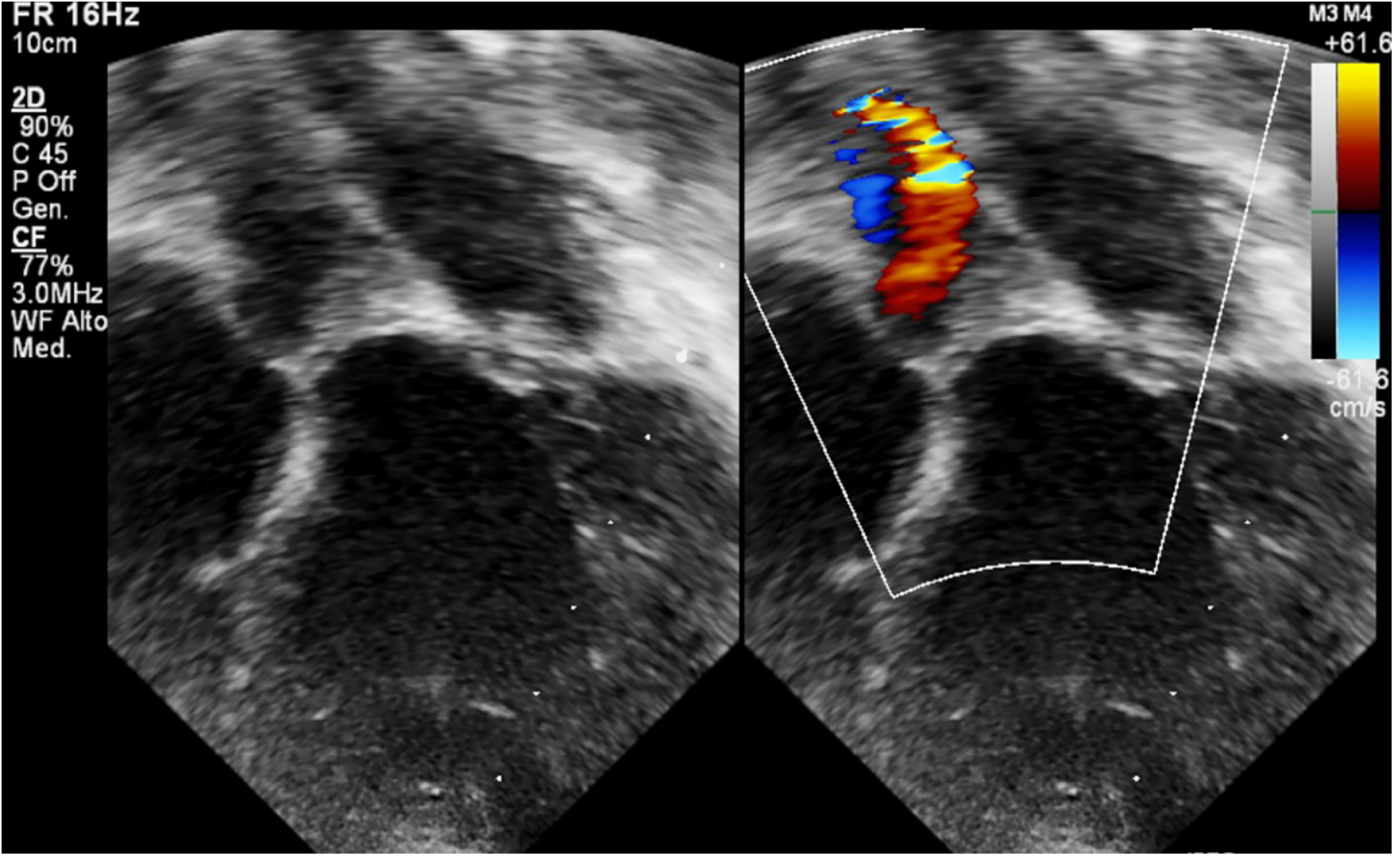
BH outflow cannula showing color Doppler flow in ascending aorta.
